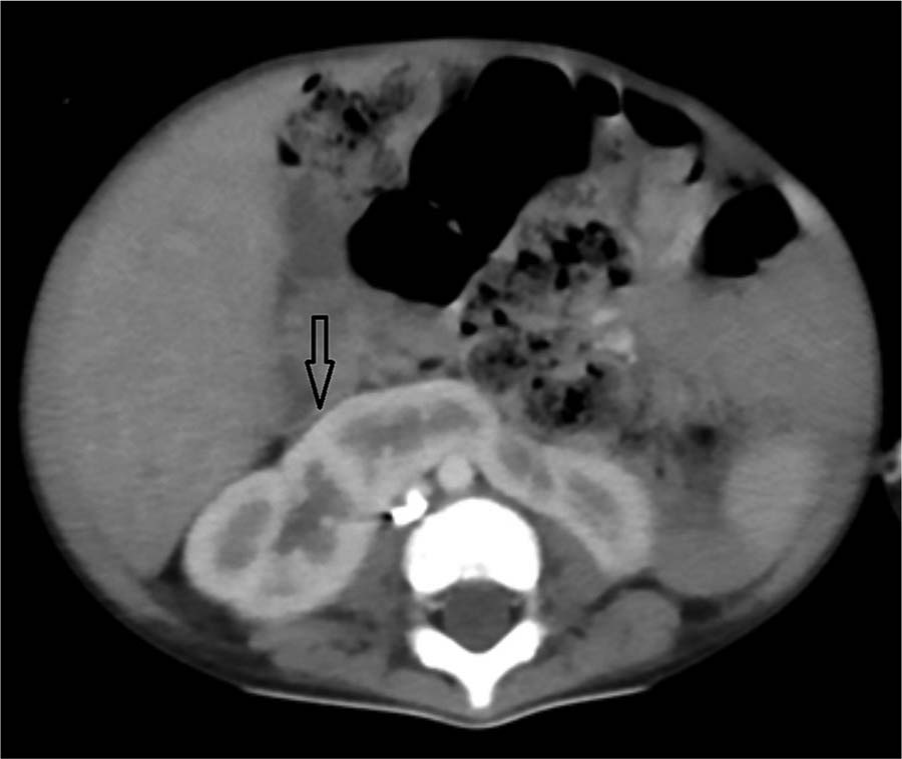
Axial abdominal CT demonstrating a horseshoe kidney.
